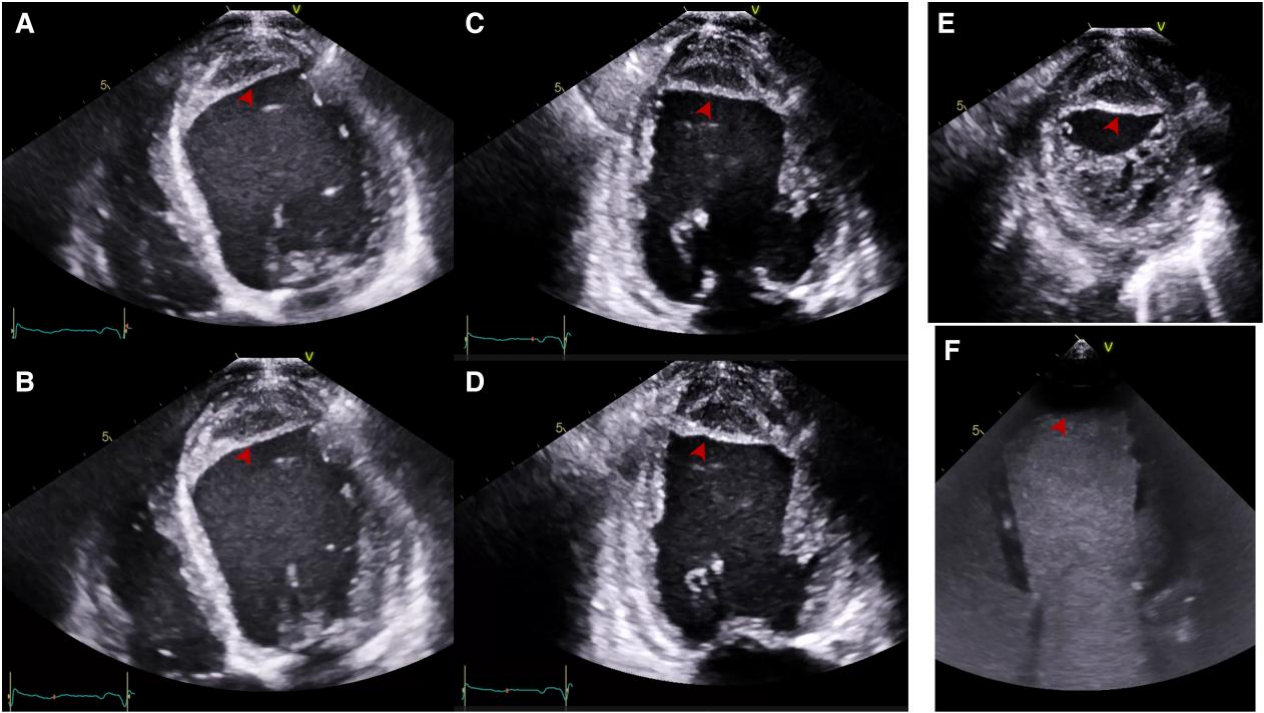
TTE in Patient 2. End-diastole (ED) and end-systole (ES) apical four-chamber (A4C) views (*A* and *B*, respectively). ED (*C*) and ES (*D*) A2C views. Note the systolic expansion of the intramyocardial neo-cavity, compatible with a large anteroapical IMDH (arrowheads). Apical short-axis (SA) view of flap-like endocardial border (*E*). Contrast TTE in A4C view (*F*) shows lack of contrast flow from the LV.
